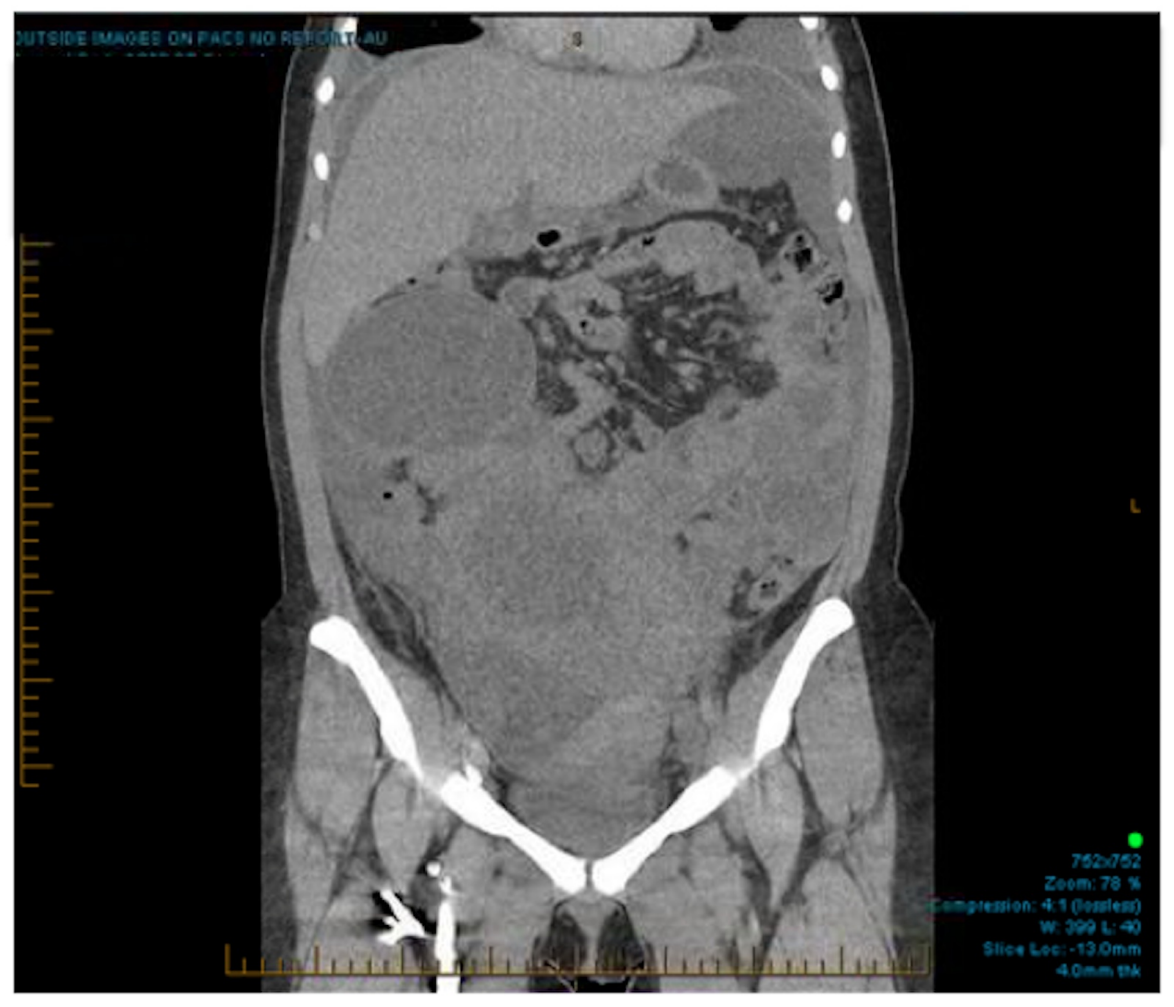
Staging CT scan. Coronal CT scan showing multiple abdominal agglomerated masses with cystic components revealing evidence of peritoneal carcinomatosis.
